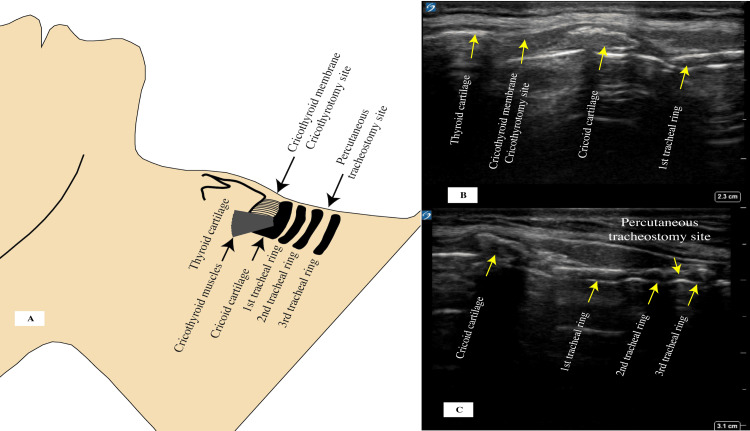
(A) Cricothyroid membrane and anatomy for cricothyrotomy and percutaneous tracheostomy. (B) Ultrasound image showing the relation of the cricothyroid membrane to the thyroid and cricothyroid cartilages with the first and second tracheal rings. (C) Cricothyroid cartilage with the sub cricoid space and the first three tracheal rings. Credit: Image A created by the authors.
